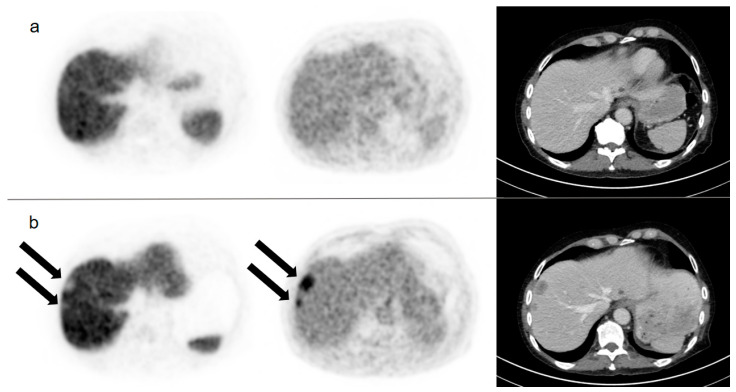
Corresponding axial slices of [^18^F]PSMA-1007 PET (first column), FDG PET (second column), and contrast enhanced CT (third column) of a 69-year old patient before PSMA radioligandtherapy (a) and after four cycles of PSMA radioligandtherapy with new FDG+/PSMA− liver metastases (black arrows); (b) fixed inverse gray-scale are displayed with SUV window setting from 0 to 20 ([^18^F]PSMA-1007 PET) and 0 to 5 (FDG PET), respectively.
